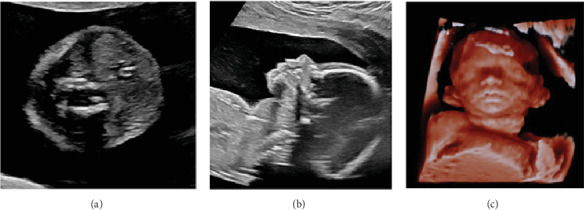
Ultrasound images of fetal goiter at 20.4 weeks gestation. Panel A,B: ultrasound 2D sagittal (A) and longitudinal (B) planes; Panel (C) 3D ultrasound.
